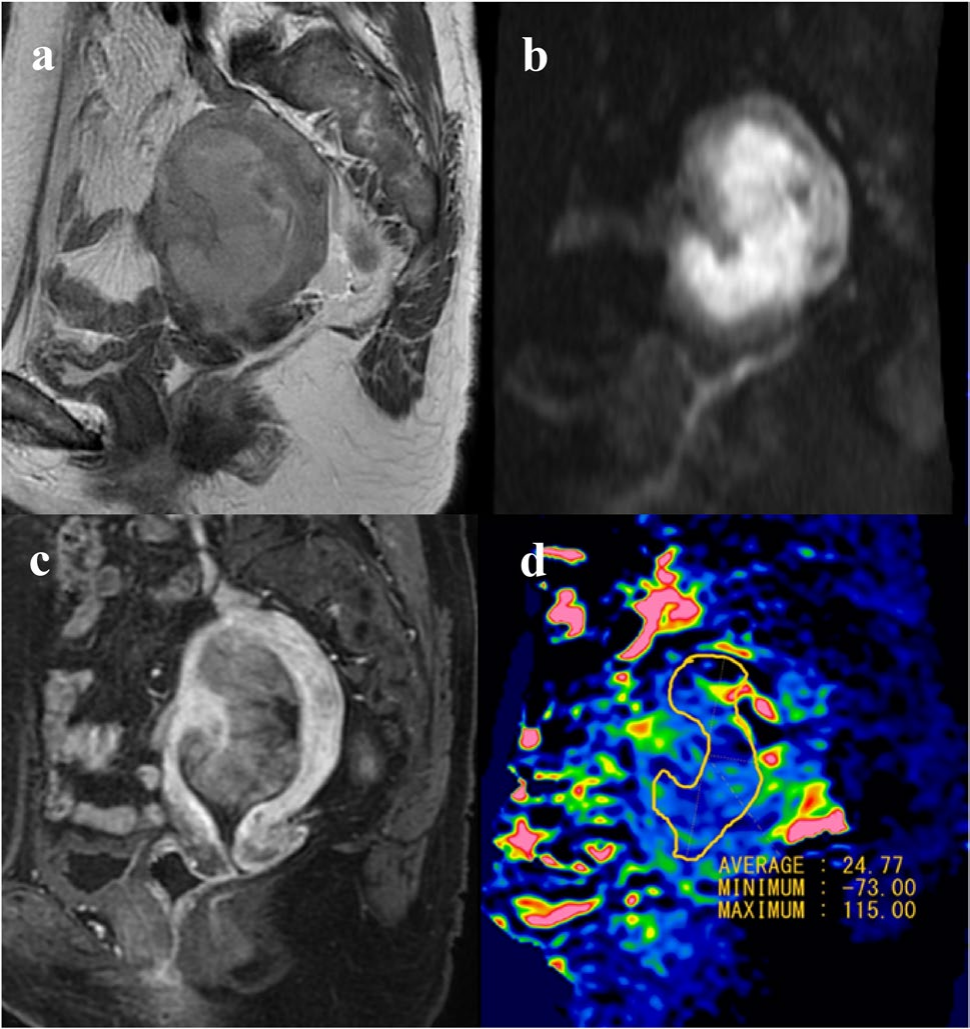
Images and placement of the ROI in a case of clear cell carcinoma. a Represents the T2WI, b represents the DWI (*b* = 800), and c represents the contrast enhanced T1WI, d represents MTR_asym_ (3.5 ppm) image. The values shown in the figure are multiplied by 1000 to obtain an integer value. Consequently, a value of 10 in the figure is 1.0%. The MTR_asym_ (3.5 ppm) image shows very high signal areas within the ROI. The APT_mean_ and APT_max_ obtained by reader 1 were 2.78% and 12.7%, respectively. The APT_mean_ and APT_max_ obtained by reader 2 were 2.48% and 11.50%, respectively. The APT signal pattern was classified as high signal by necrosis
